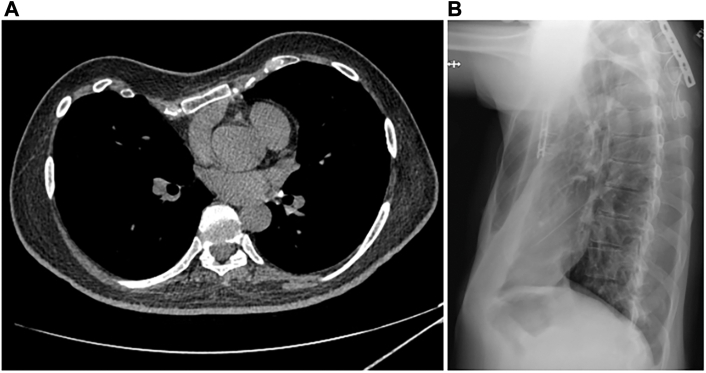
Preoperative imaging. (A) Computed tomography scan showing loss of sternal projection. (B) Chest radiograph showing pectus excavatum.
